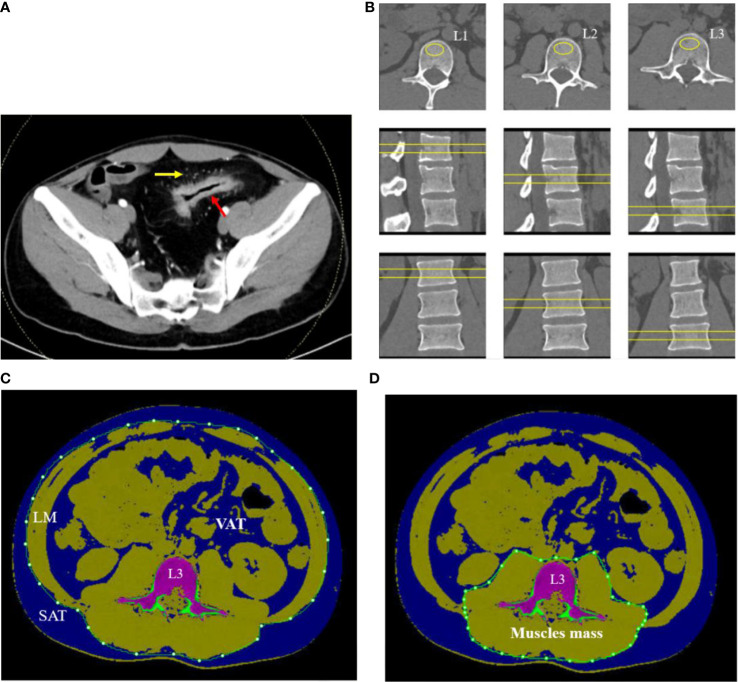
A 32-year-old man with CD. (A) Contrast-enhanced CT scan of small intestine showed bowel wall thickening, rich blood supplying (comb sign). (B) The L1–L3 BMDs were measured by QCT on axial, coronary, and sagittal plane. (C) The SAT, VAT, and LM were manually drawn around the abdominal wall at the level of L3 midplane. (D) Muscle mass around lumbar vertebrae were measured within the range manually drawn around the fascial borders of muscles (psoas and paraspinal muscles) at the level of L3.
